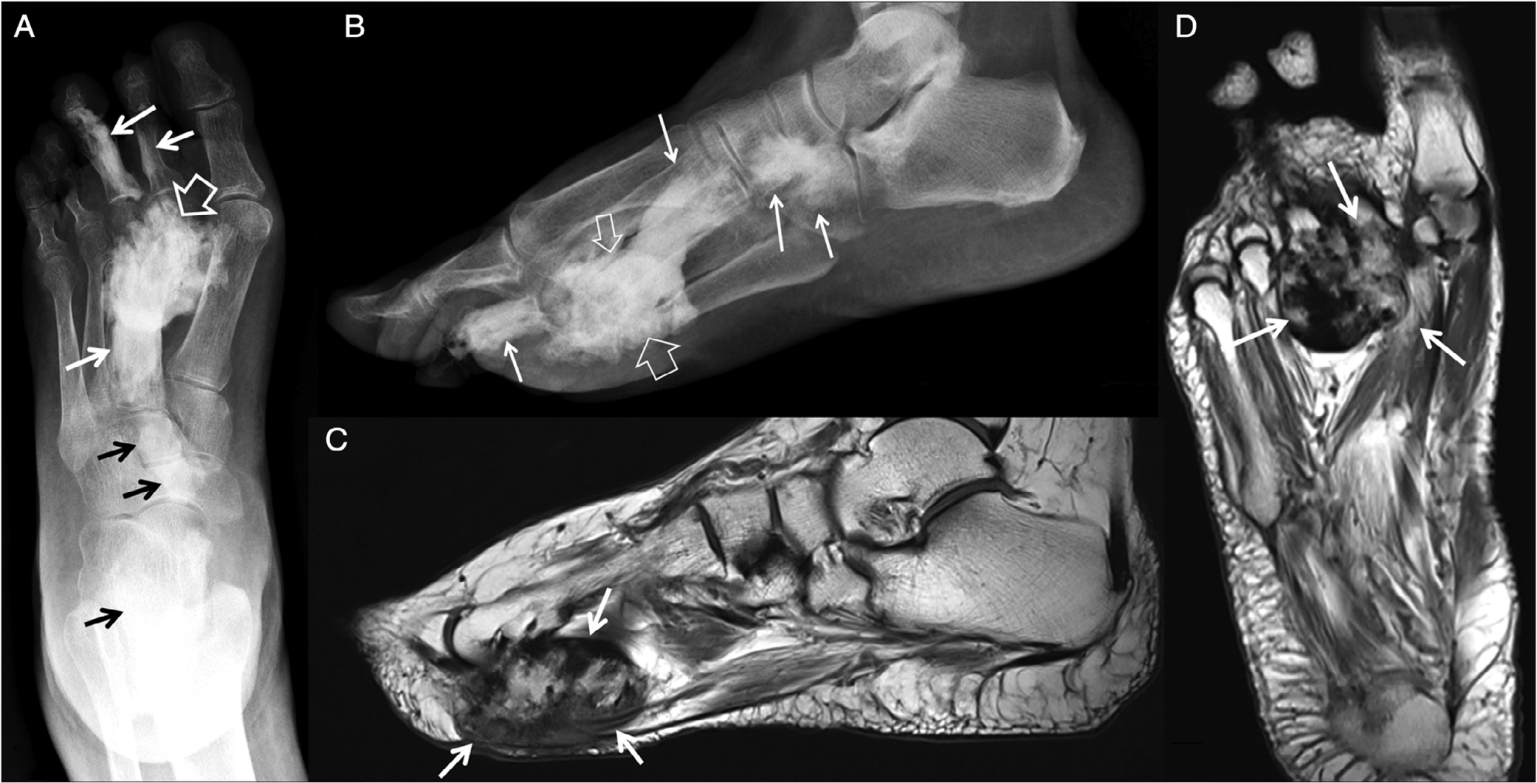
Various foot lesions in melorheostosis. A 63‐year‐old female patient (Melo‐41) showing on plain anteroposterior (*A*) and lateral (*B*) plain X‐Rays, endosteal hyperostosis lesions in the second proximal phalanx, in the third proximal and middle phalanx, in the third metatarsal, in the middle cuneiform and navicular bones in the midfoot, and distal tibia, and proximal talus (arrows). A large ossified lesion projects over midfoot and metatarsal bones (open arrows). Sagittal (*C*) and axial (*D*) T1‐weighted MR images show the location of the large soft tissue ossification plantar to distal metatarsals, displacing the muscles of the plantar aspect (arrows) in greater detail in comparison with plain radiographs.
